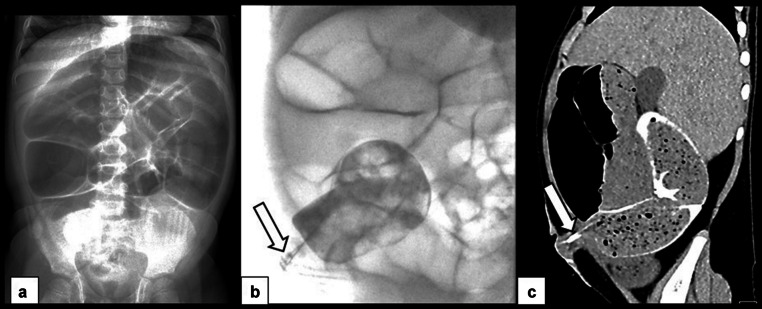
Abdominal radiographs, fluoroscopy performed through the ileostomy, and CT with IV and enteric contrast injected through the ileostomy in a 5-year-old boy with MMIHS who presented with emesis and decreased ostomy output. This patient had a history of ostomy revision due to fascial tightness at the skin soon after his ileostomy. (a) Frontal abdominal radiograph shows marked dilation of multiple bowel loops. (b) AP fluoroscopic image with contrast injected through the ostomy demonstrates similar findings of a narrow channel through the abdominal wall at the site of ileostomy (*arrow*). (c) Sagittal CT abdomen and pelvis with enteric contrast injected through the ileostomy shows persistent narrowing of the ostomy as it traverses the abdominal wall (*arrow*), with severe upstream dilation of bowel loops. This patient was managed with daily catheter decompression through the ostomy and was thought to have a partial obstruction at the level of the ostomy. A follow-up frontal abdominal radiograph (not shown) showed decreased dilation of bowel with bowel decompression through the ostomy, and the patient was eventually transitioned to a regular diet
